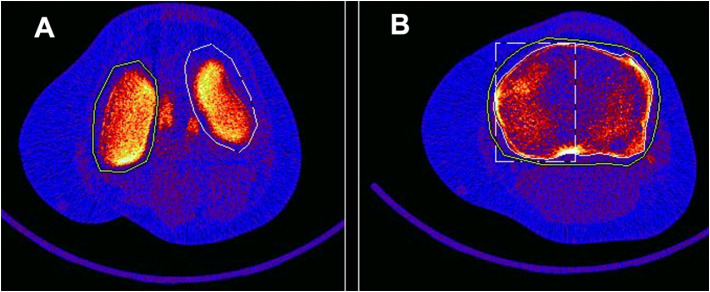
Example of femoral condyle and tibia regions of interest from a peripheral quantitative computed tomography image.
